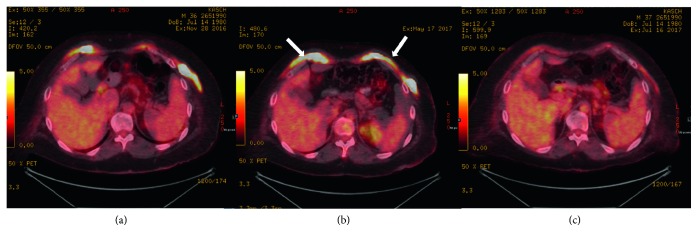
Series of FDG-PET/CT images of the chest at the level of T11 throughout the same period. (a) Initial PET scan at the time of presentation (November 2016). (b) Progression of lower anterior chest wall involvement (arrows) (May 2017). (c) Interval regression of lower anterior chest wall involvement after starting vemurafenib (July 2017).
